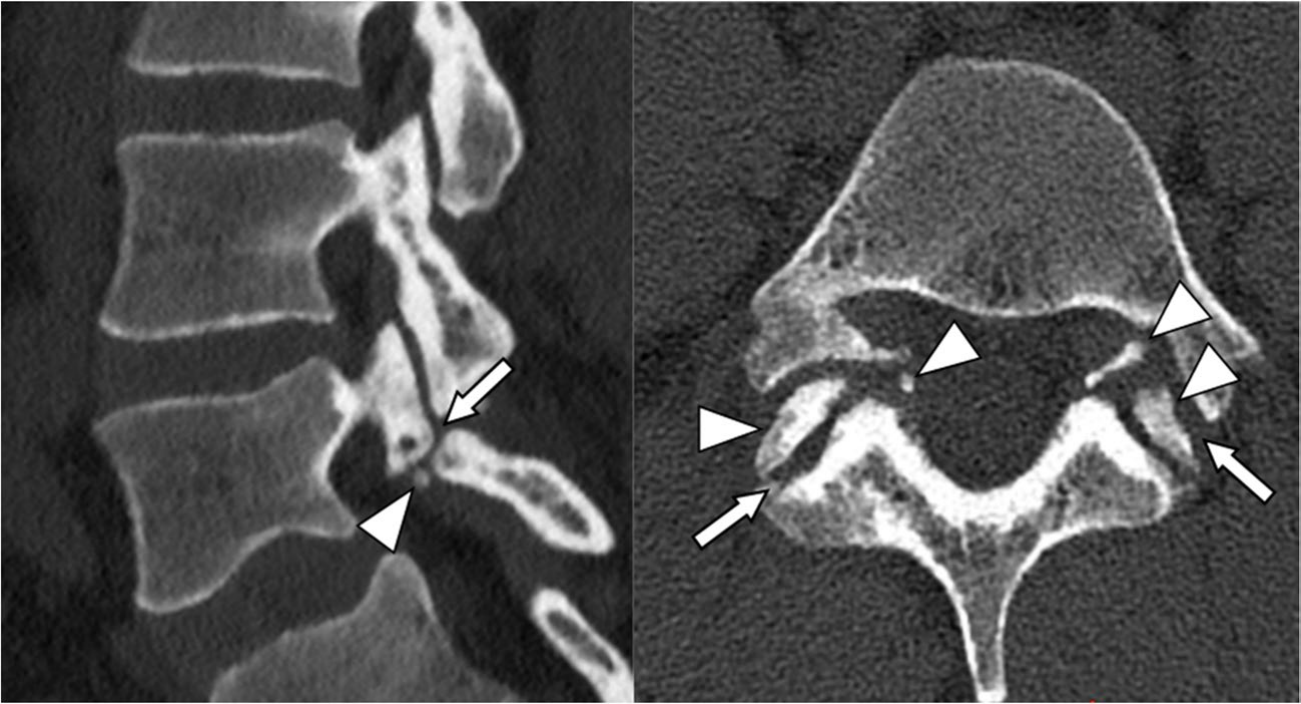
Sagittal left follow-up CT image of the lumbar spine and axial image of a persisting lysis gap (arrow) on the left and right side and multiple ossicles in the lysis gap (arrowheads) in a 23-year-old female patient
